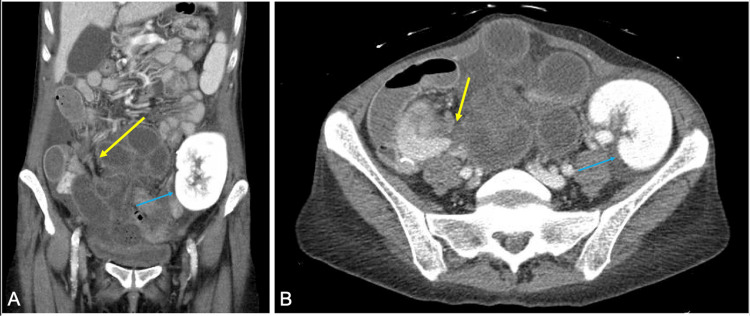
(A) Coronal and (B) axial views of CT of the abdomen and pelvis showing the transition point of a closed-loop small bowel obstruction. Yellow arrow: adhesive band; Light blue arrow: renal allograft.
